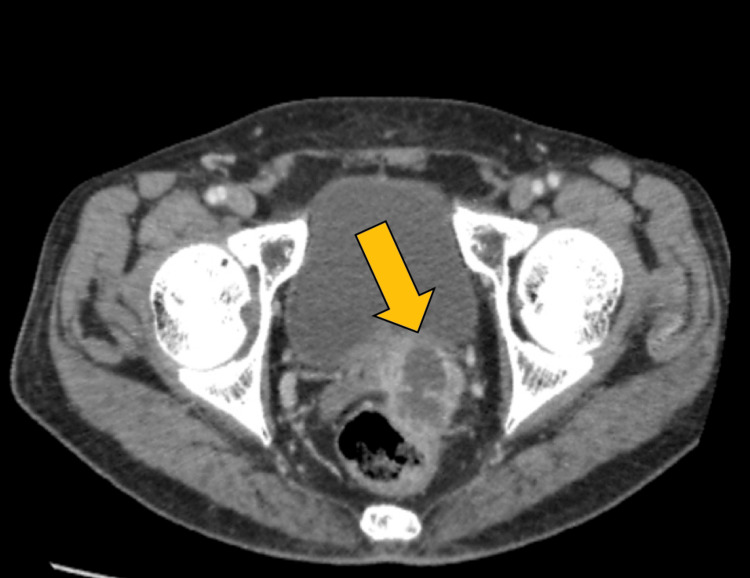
Axial CECT scan showing the cyst with internal echoes in the left seminal vesicle CECT: Contrast-enhanced computed tomography.
